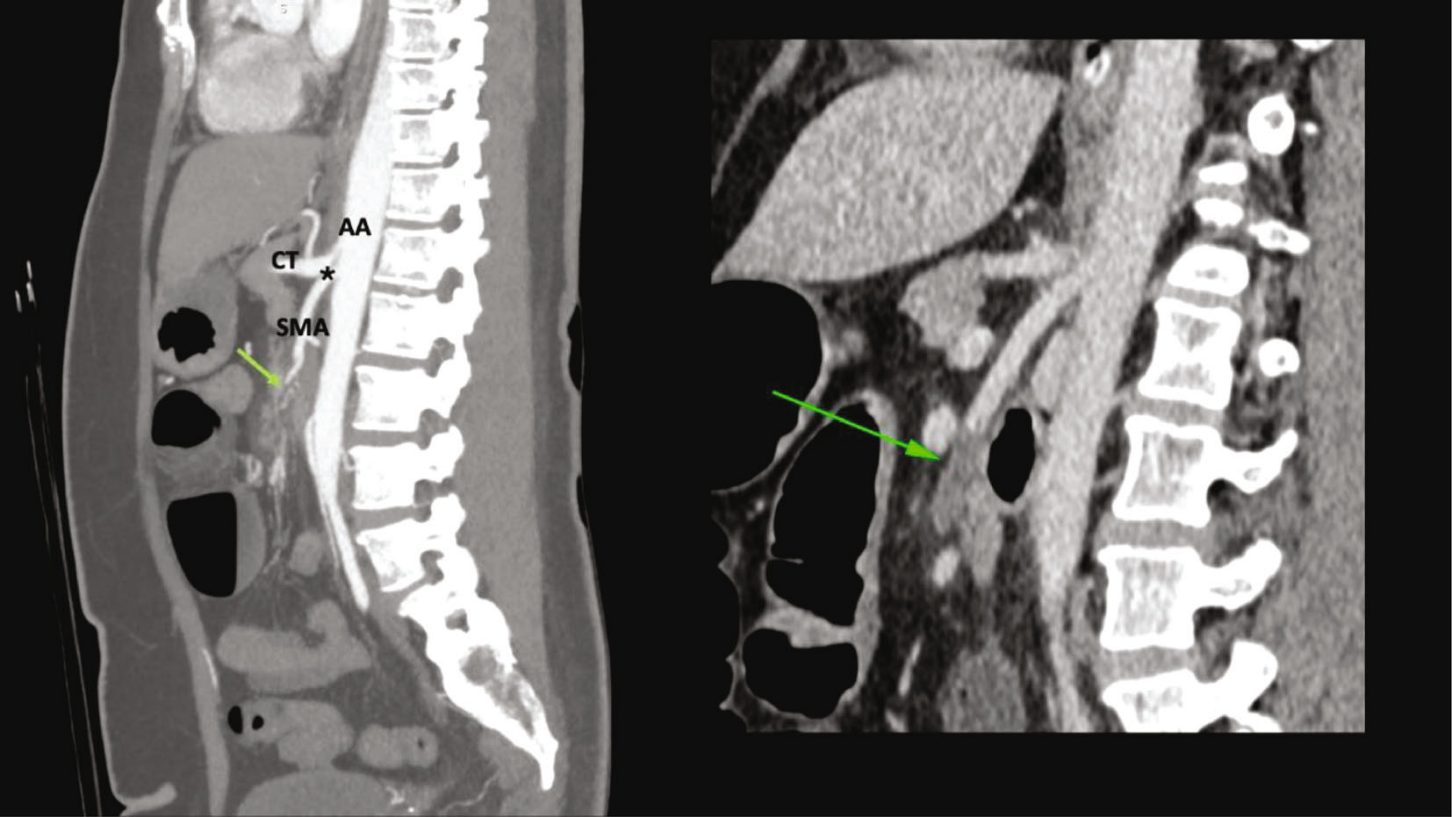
Sagittal view of a CT mesenteric angiogram showing a short celiac-mesenteric trunk (asterisk) arising from the abdominal aorta. The trunk further branches into the celiac trunk (CT) and superior mesenteric artery (SMA). Inset: an intraluminal thrombus can be seen within the SMA appearing as a filling defect (green arrow) and causing flow cessation in the lumen.
